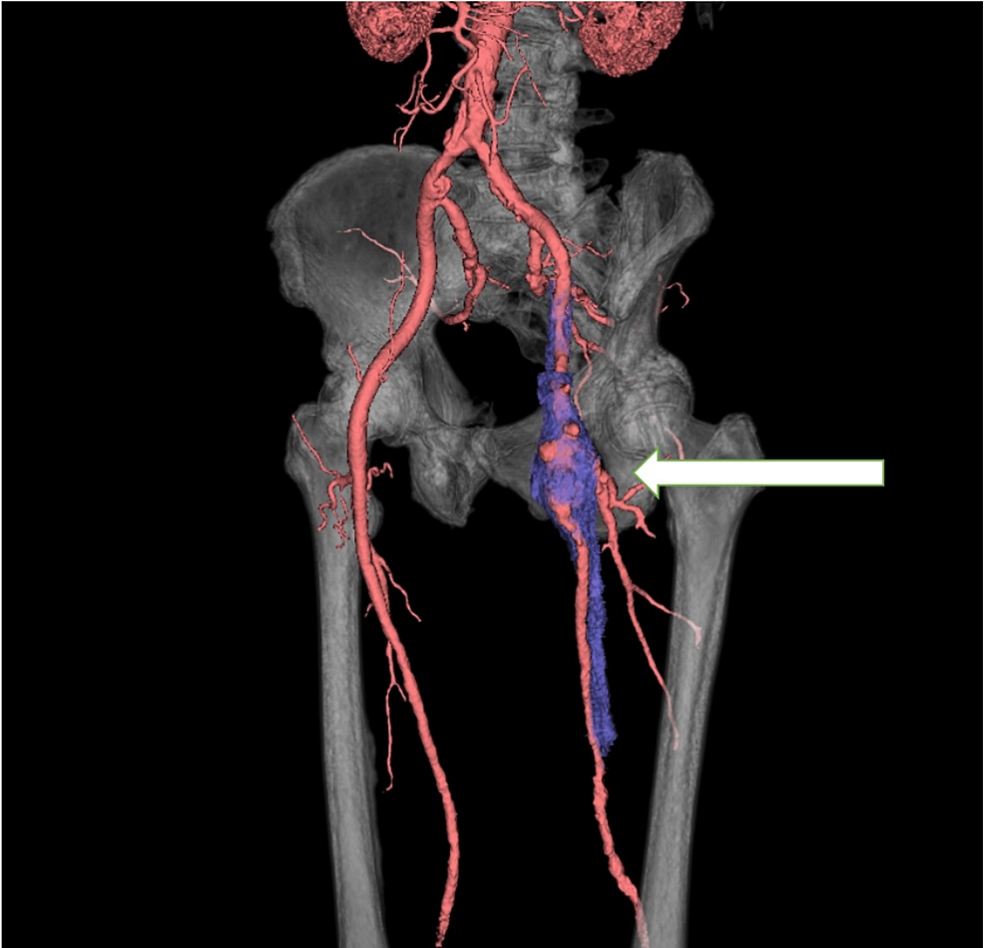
3D constructed image of preoperative CT Irregular widening from the left CFA to SFA and DFA (⇦). CT - computed tomography; CFA - common femoral artery; SFV - superficial femoral vein; SFA - superficial femoral artery; DFA - deep femoral artery
